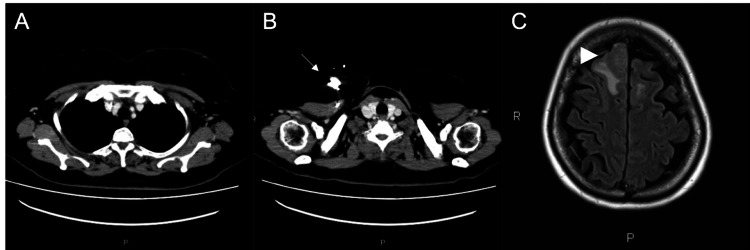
Imaging findings after six cycles of TP-TAM CT scan (A, B) and MRI of the brain (C). The white arrow points to the central venous port. The arrowhead points to the largest of the multiple brain metastases. TP-TAM: Trastuzumab + pertuzumab + tamoxifen; CT: Computed tomography; MRI: Magnetic resonance imaging
